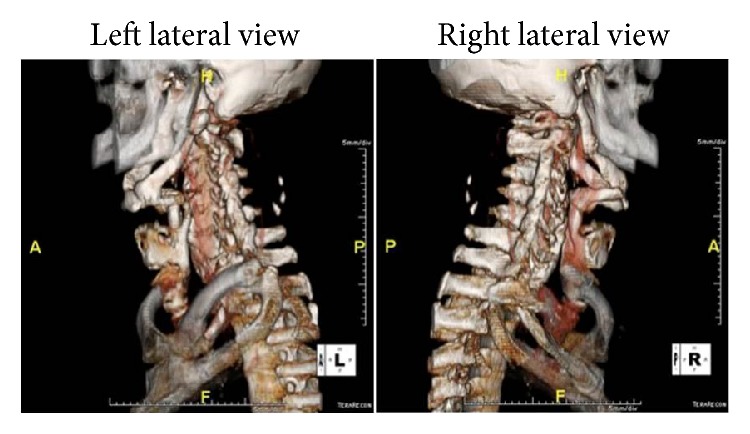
CT scan of the neck with 3-dimensional reconstruction of the larynx.
